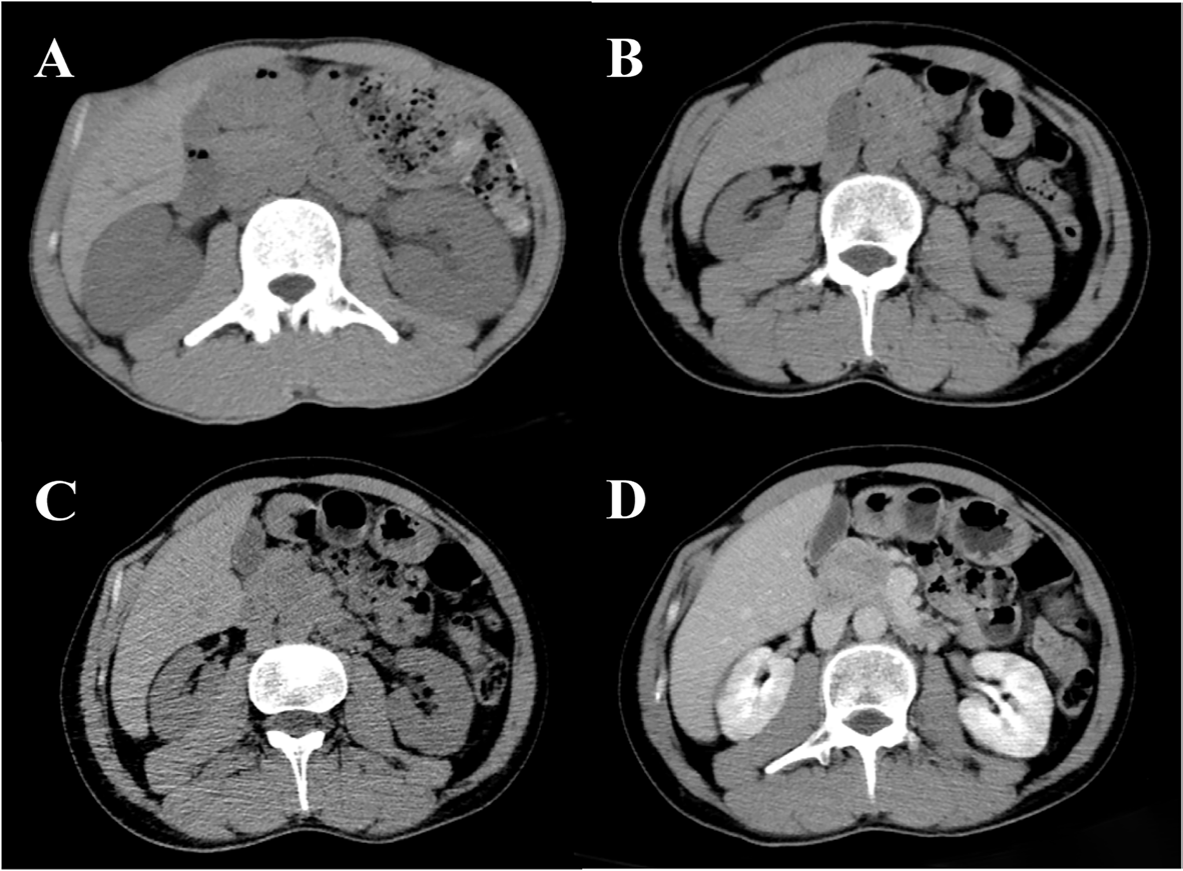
Abdominal computed tomography of Case 3. A August 2020, B November 2020, C Jaunary 2021, D Jaunary 2021 (enhanced)
